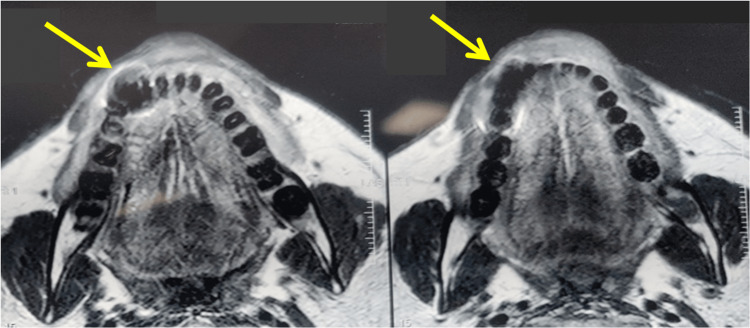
MRI showed a hypointense area of 42, 43, and 44 regions.
